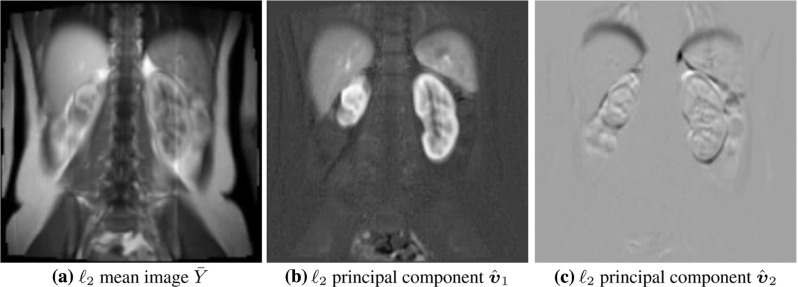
a Mean image and b–c the first two principal components of the DCE-MRI sequence obtained by $$\ell _2$$ methods. Intensity changes in the image sequence associated with contrast agent and with physiological motion are conspicuously apparent in the components $${\hat{{{\varvec{v}}}}}_1$$ and $${\hat{{{\varvec{v}}}}}_2$$, respectively
